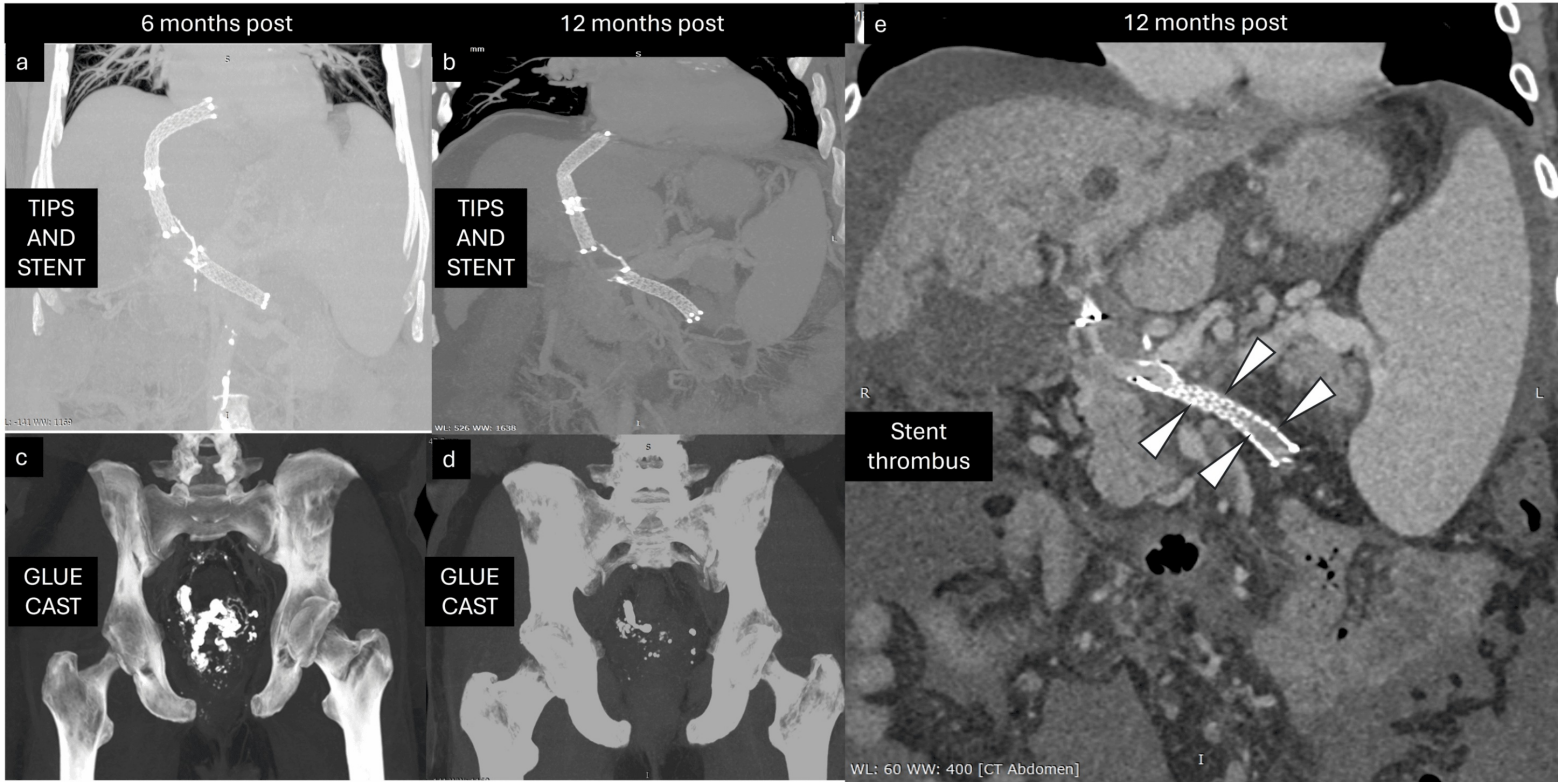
Follow-up contrast computed tomography images Images from bi-annual follow-up computed tomography: (a) and (c) are six months post-CT images showing expected positions of the stent and glue cast, respectively. (b) and (d) are 12 months post-CT images showing a slightly deformed superior mesenteric vein (SMV) stent. (e) Deformed SMV stent with distal thrombus (white arrowheads).
